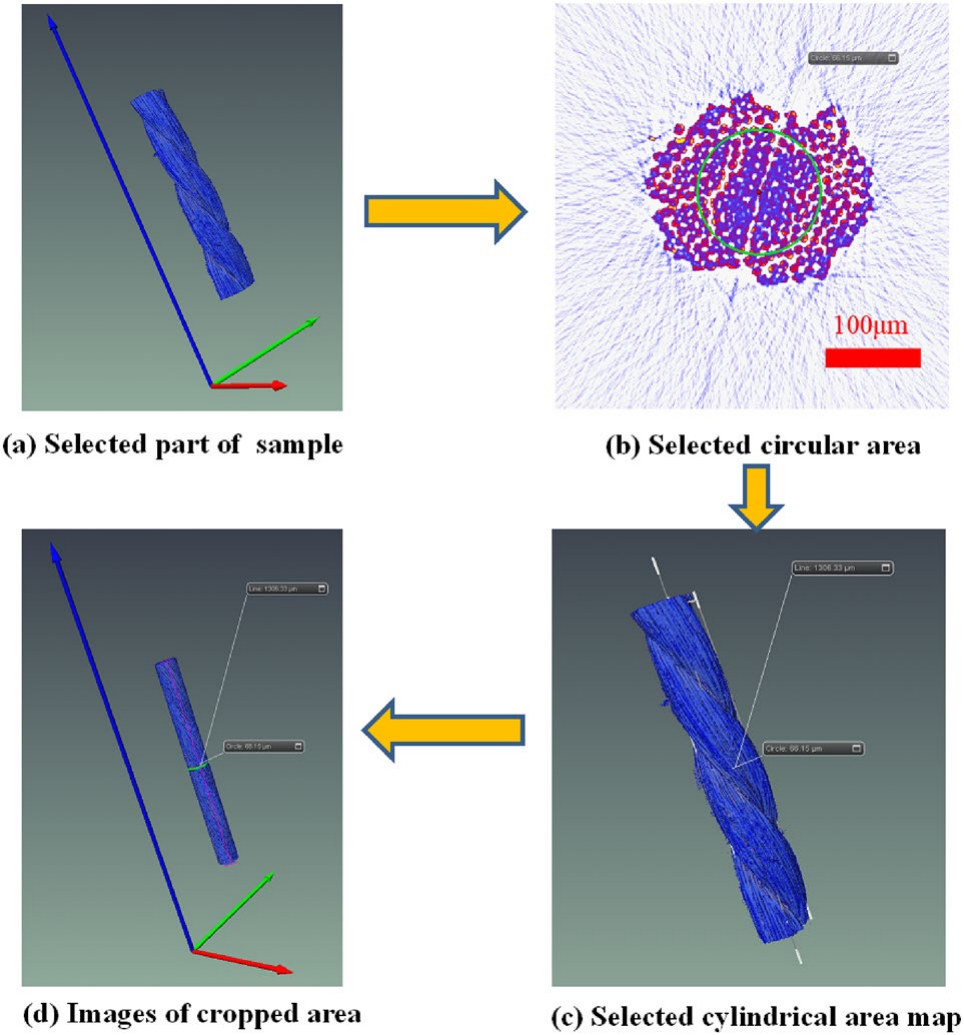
Area selection from the nylon CT images.
